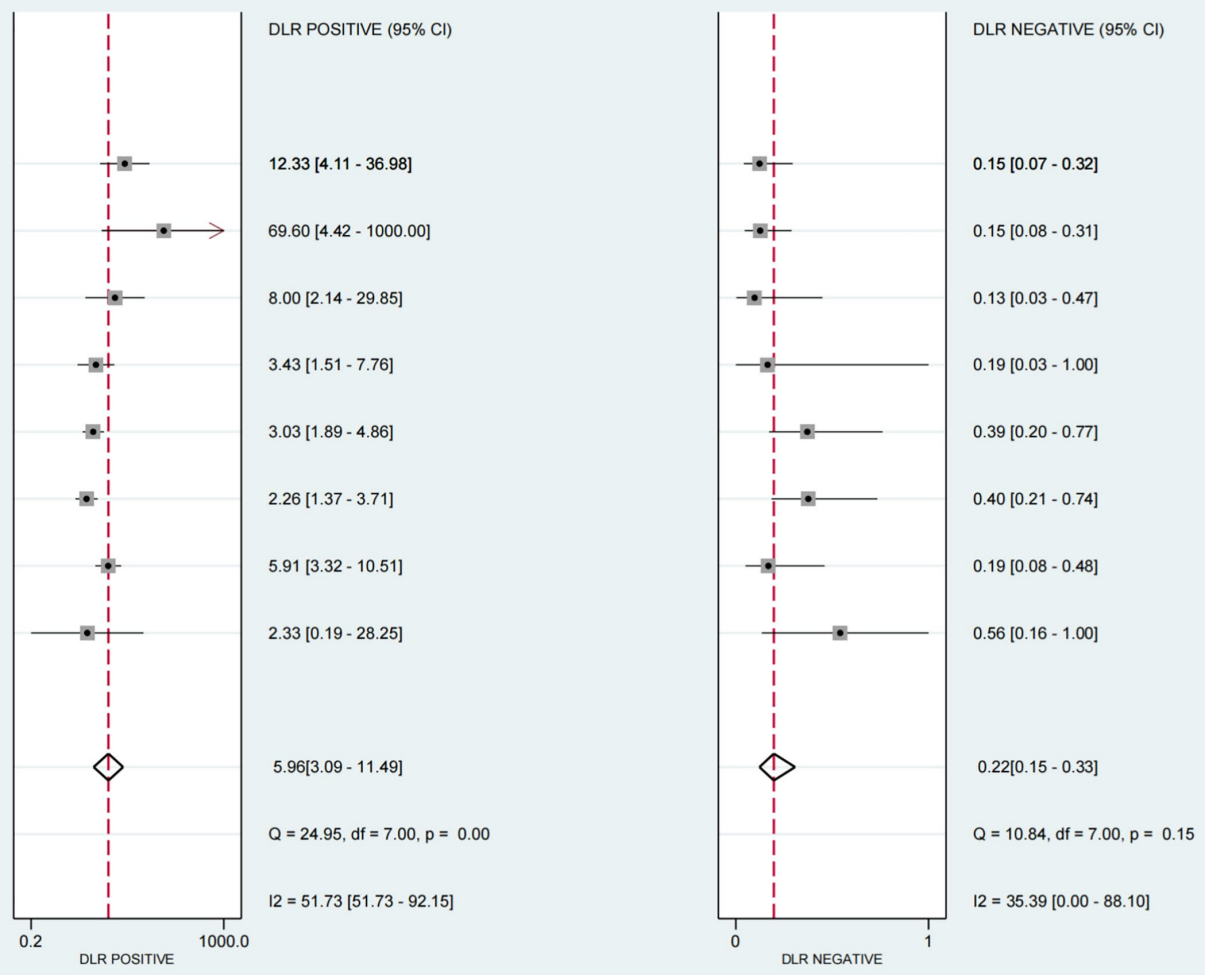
Likelihood of individual studies in ultrasound-based dysphagia diagnosis.
